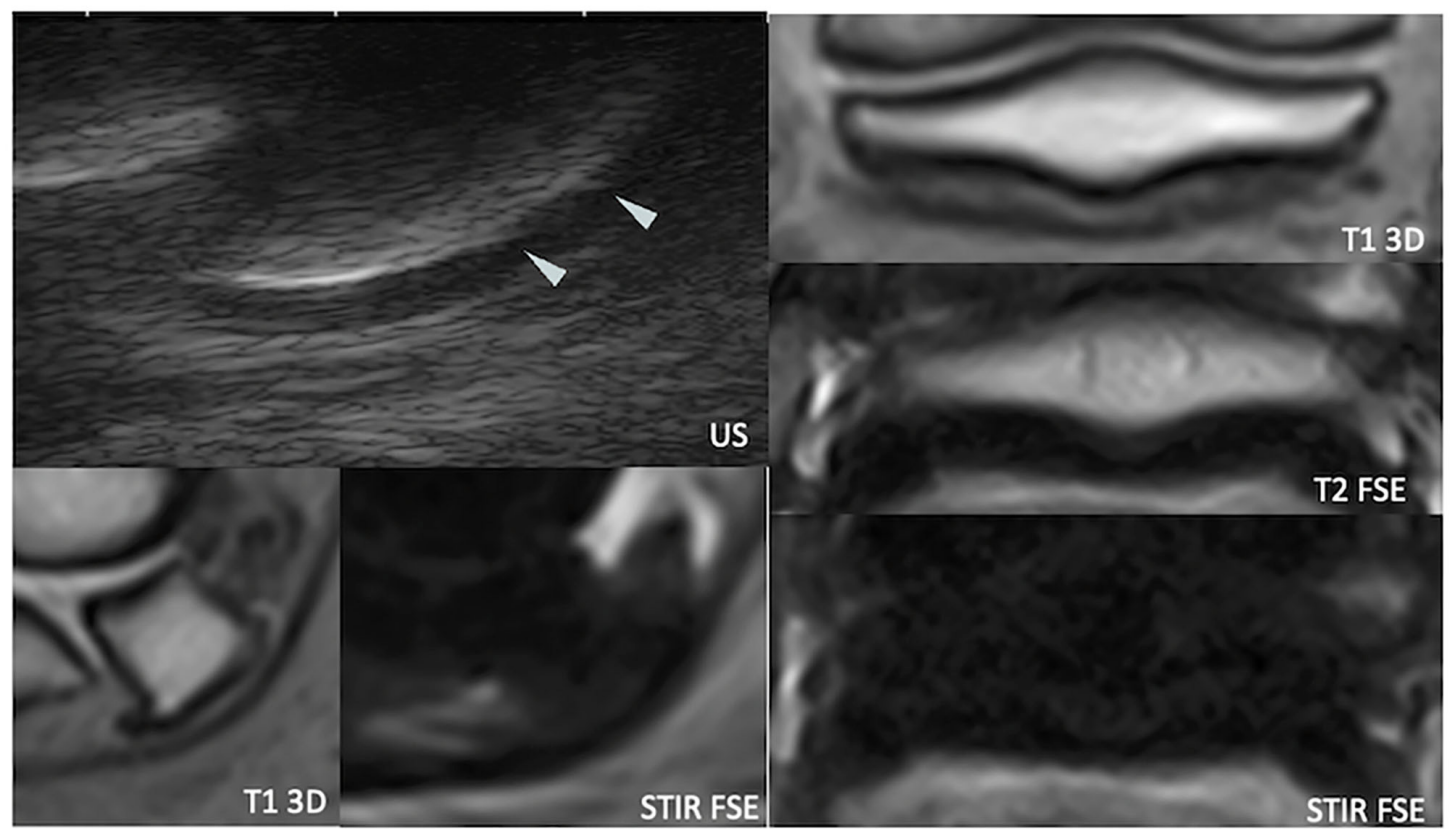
Longitudinal transcuneal ultrasonographic (top left), sagittal (bottom left), and transverse (right) magnetic resonance (MR) images of a right front foot, focused on the navicular bone. The ultrasonographic image has been flipped vertically to obtain the same orientation than the MR images. The mid-sagittal fossa of the palmar navicular compact bone shows sharp ultrasonographic margins (arrowheads). US, ultrasonography; T1 3D, tridimensional gradient echo T1 weighted sequence; T2 FSE, fast spin echo T2 weighted sequence; STIR FSE, fast spin echo short tau inversion recovery weighted sequence.
